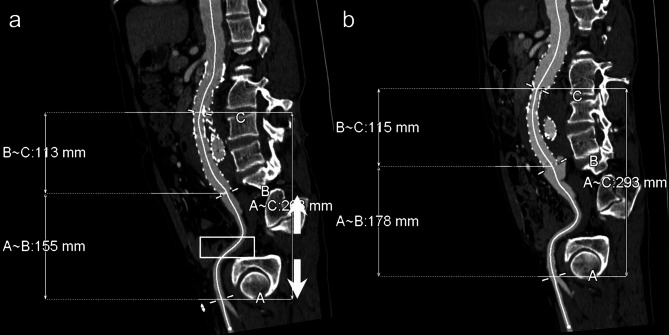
Assessment of limb migration length in a 63-year-old male patient with an infrarenal abdominal aortic aneurysm following endovascular aortic repair. (a) The initial post-operative CTA scan, performed one week after the procedure, measured the distance from the origin of the deep femoral artery to the distal end of the left iliac limb stent-graft as 155 mm. (b) At the 3-year and 8-month follow-up, the distance was measured at 178 mm on the CTA. The limb migration length was determined to be 23 mm. *CTA* computed tomography angiography.
